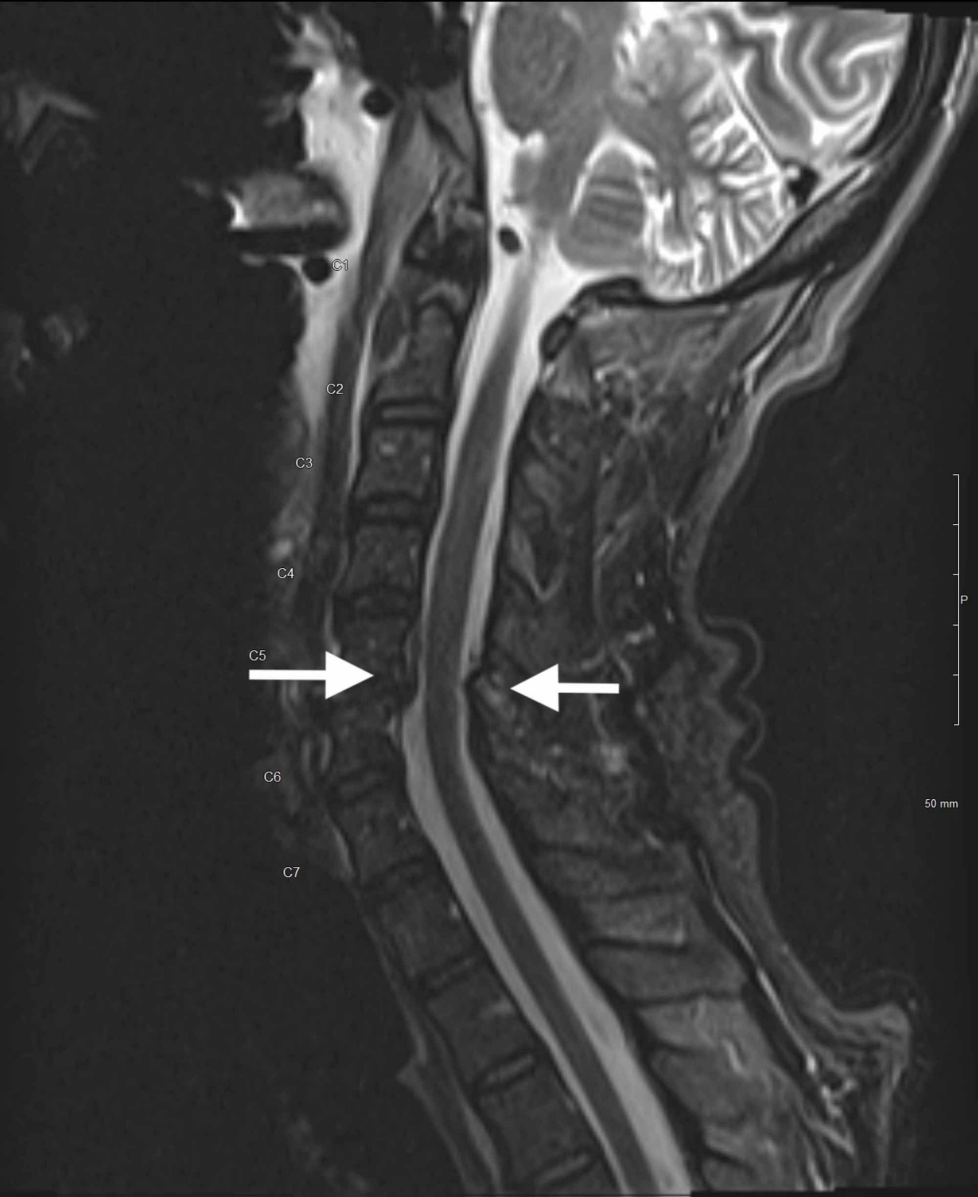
magnetic resonance imaging of the cervical spine without contrast demonstrating cervical stenosis at C5-6 level with prevertebral fluid and edema (white arrows) consistent with Froin’s syndrome.
